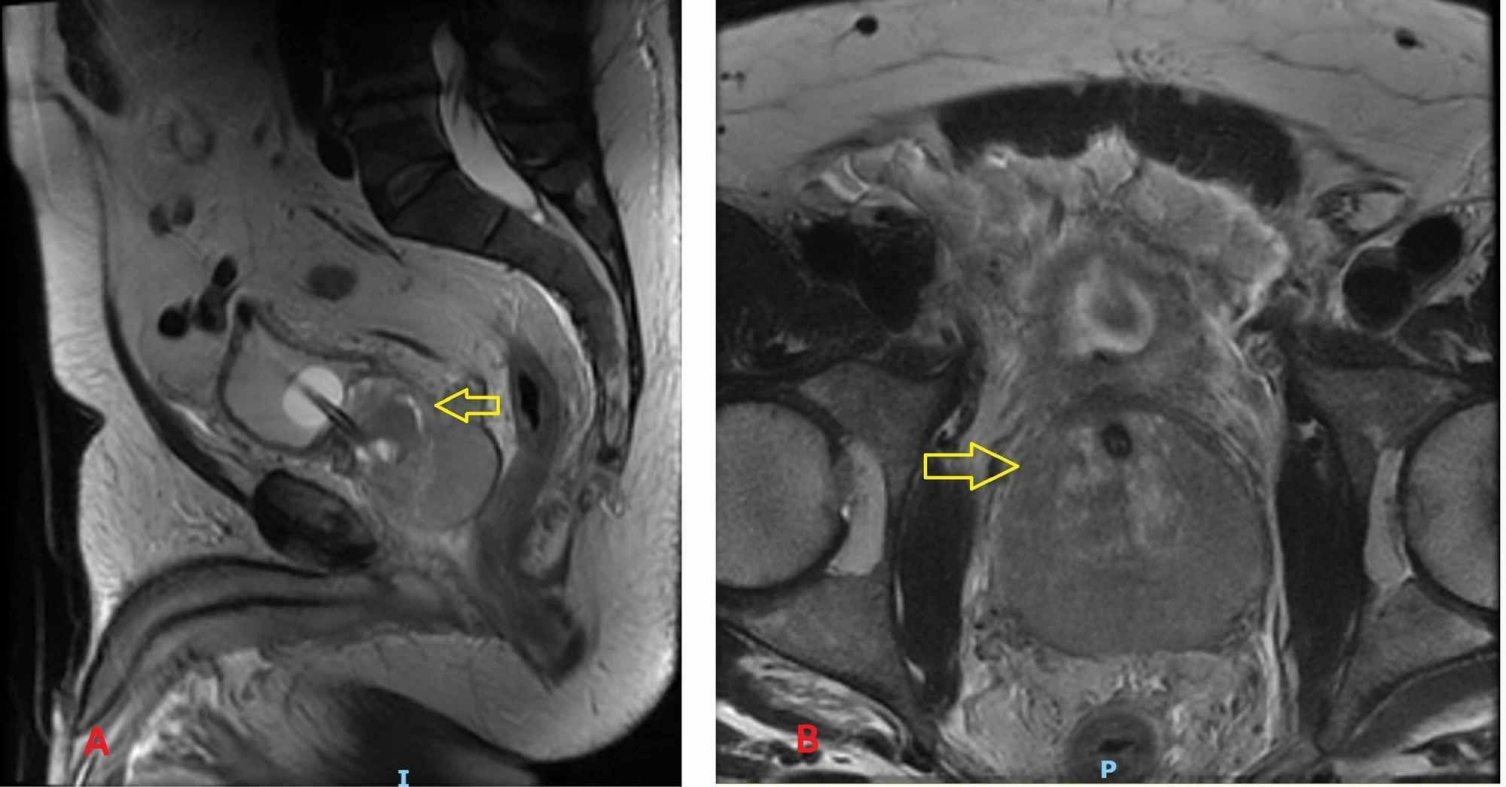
Sagittal (A) and axial (B) T2 MRI images showing marked enlargement of the prostate gland, most notably the peripheral zone with marked T2 hypointensity. Findings were concerning for prostatitis.
